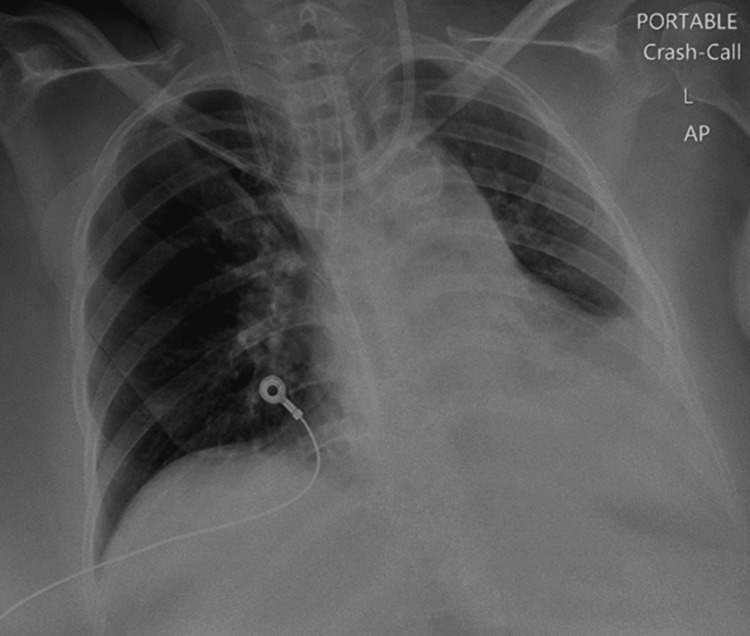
Chest X-Ray The chest X-ray revealed that the endotracheal tube was positioned low in the right main bronchus, necessitating adjustment, while the left costophrenic angle was obscured by the cardiac shadow. No airspace opacities or pneumothorax were identified.
